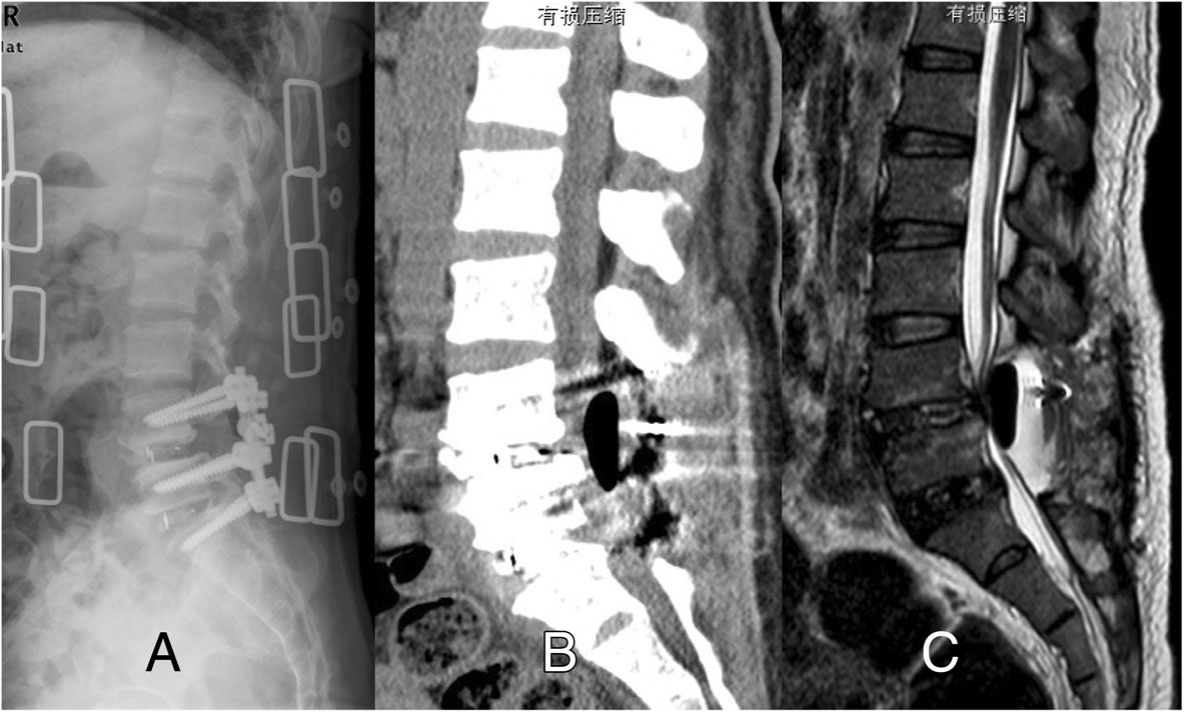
Postoperative lateral X-ray of lumbar spine after surgery revealed a black shadow in the upper surgical region (a). The patient had no comfort and no intervention was taken. 10 days later, she began to have dysfunction of excretion. CT and MRI (b and c) revealed a collection of epidural gas pseudocyst, compressing the spinal cord
